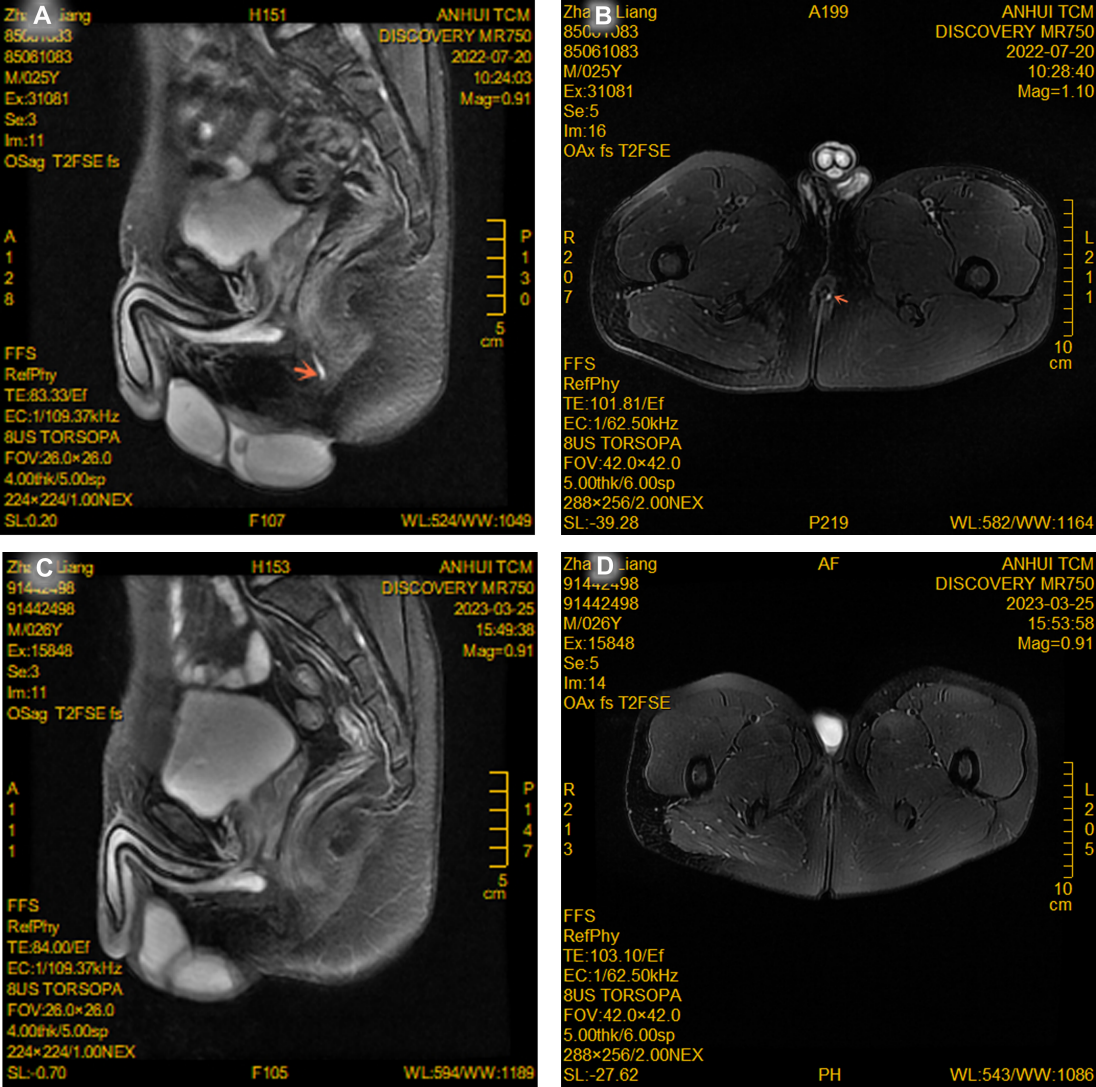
Magnetic resonance imaging of a young male before (A,B) and after (C,D) receiving NiTi-AFC internal orifice closure. The T2 phase showed that the fistula shadow with a high signal tended to disappear (red arrow point).
